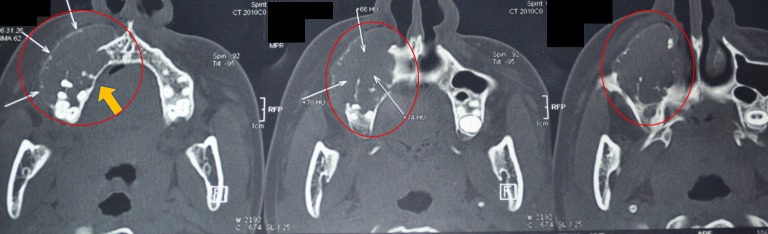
Computed tomography axial section shows expansion of the buccal cortex showing anteroposterior and labio-palatal extension (areas circled in red, denoted by the yellow arrow).
